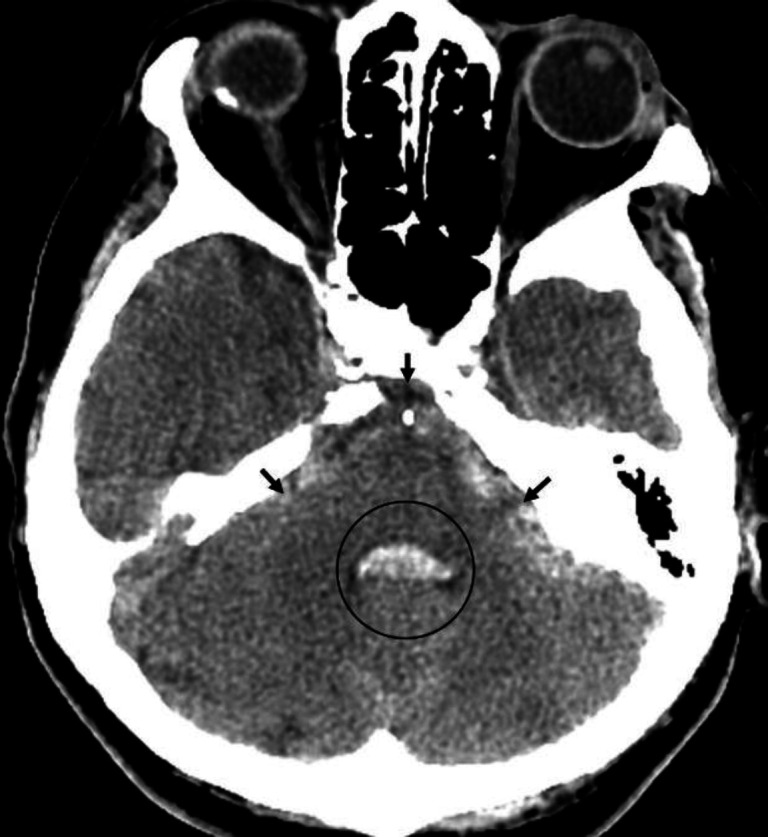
Non-contrast CT brain of a 68 year-old male with sub-arachnoid haemorrhage. Acute blood appears as a hyperdensity in the basal cisterns (black arrows). Note that the 4th ventricle (black circle) is hyperdense, indicating intraventricular extension of the haemorrhage
